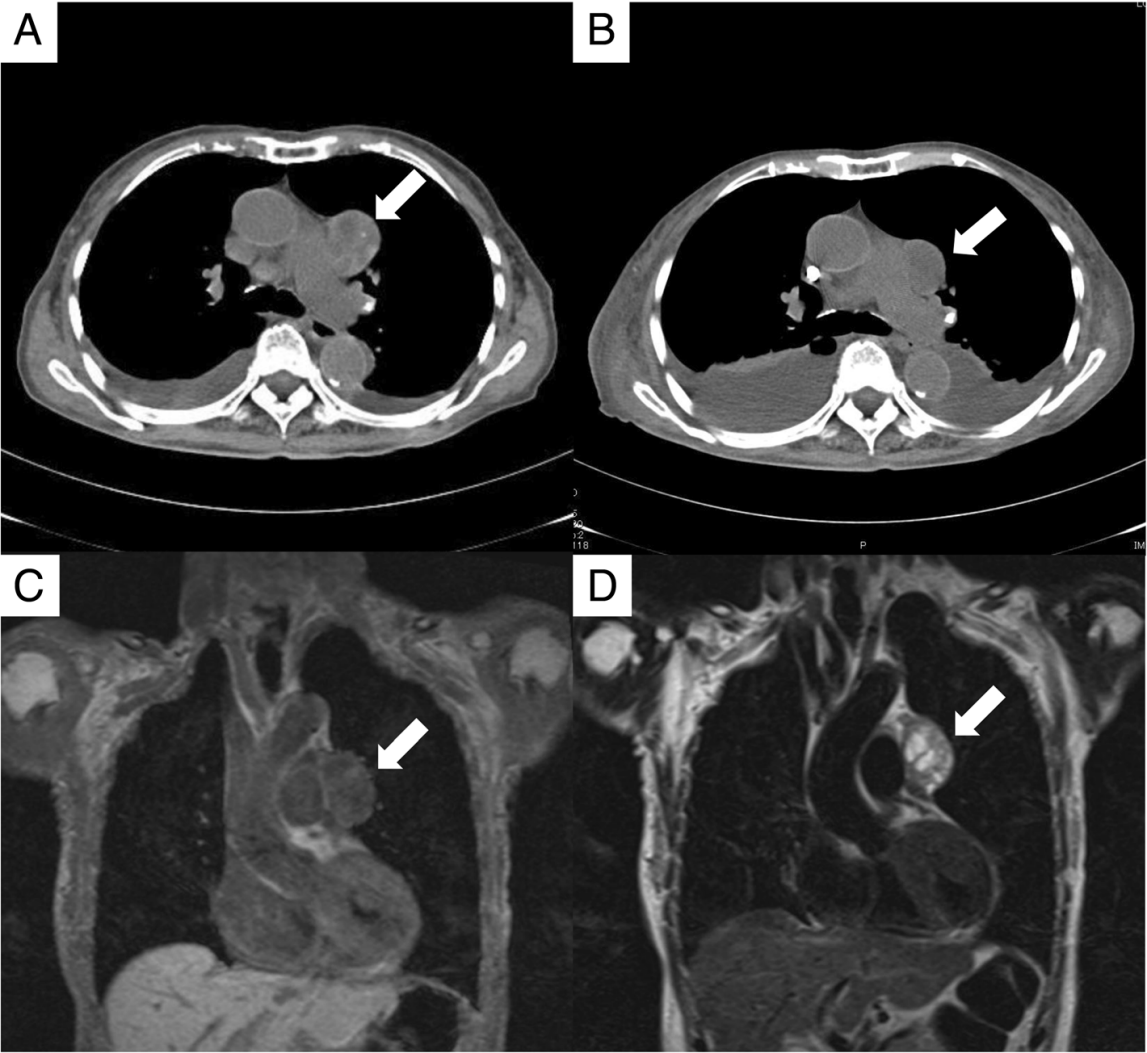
Findings of radiological examinations in the present case. a Chest CT. b Chest CT 2 months after the treatment. c Chest MRI, T1-weighted imaging coronal section. d Chest MRI T2-weighted imaging coronal section. *Arrows* indicate the lobulated tumor in the anterior mediastinum
